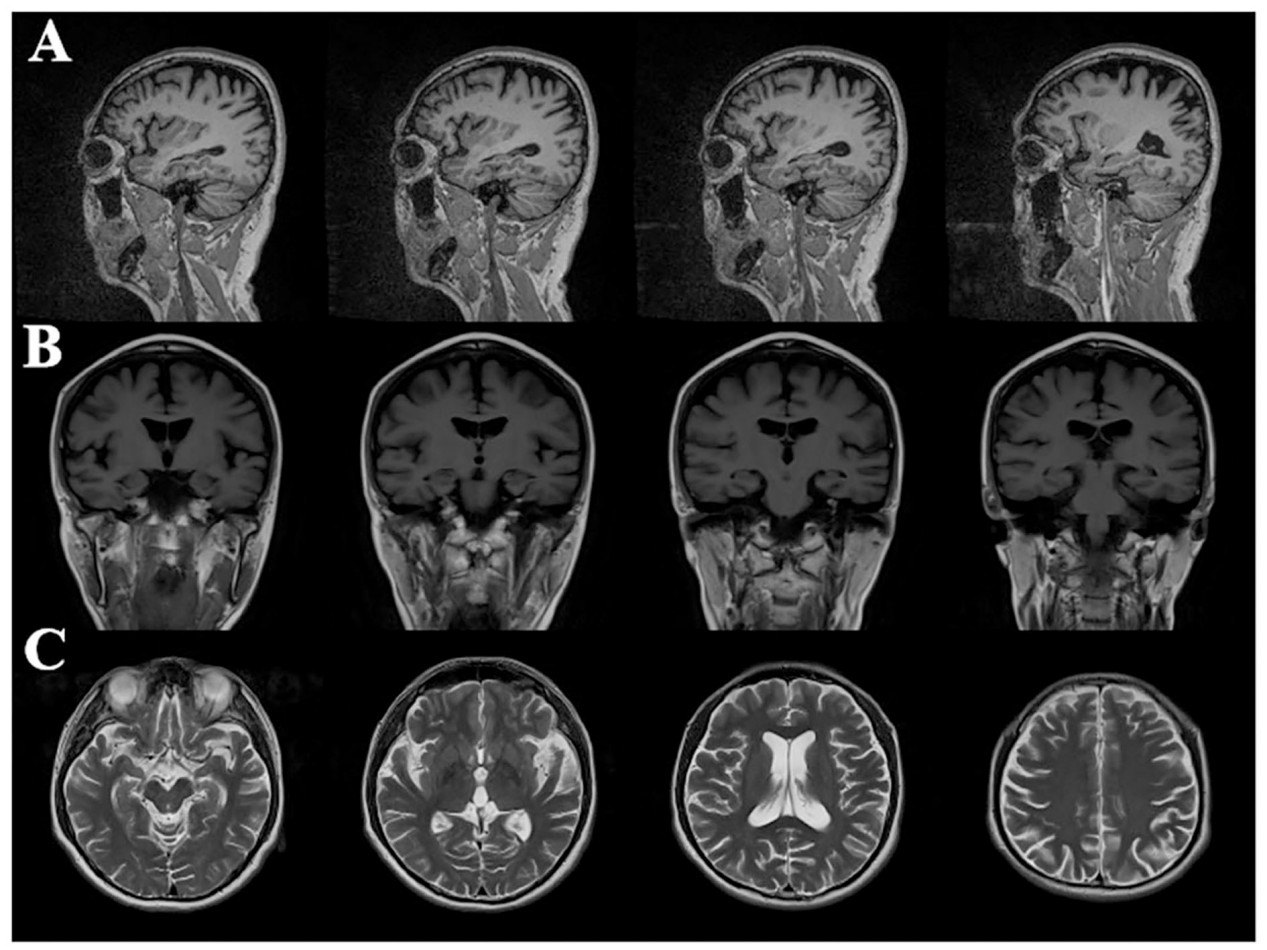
Cranial magnetic resonance imaging in patients with Alzheimer's disease: (A) sagittal image of T2 fluid attenuated inversion recovery (T2 FLAIR); (B) coronal image of T1-weighted image (T1WI); (C) cross-sectional sequences of T2-weighted image (T2WI); imaging results showed atrophy of bilateral temporal parietal lobe and posterior cingulate gyrus, and dilatation of ventricle, cistern, fissure, and sulci.
